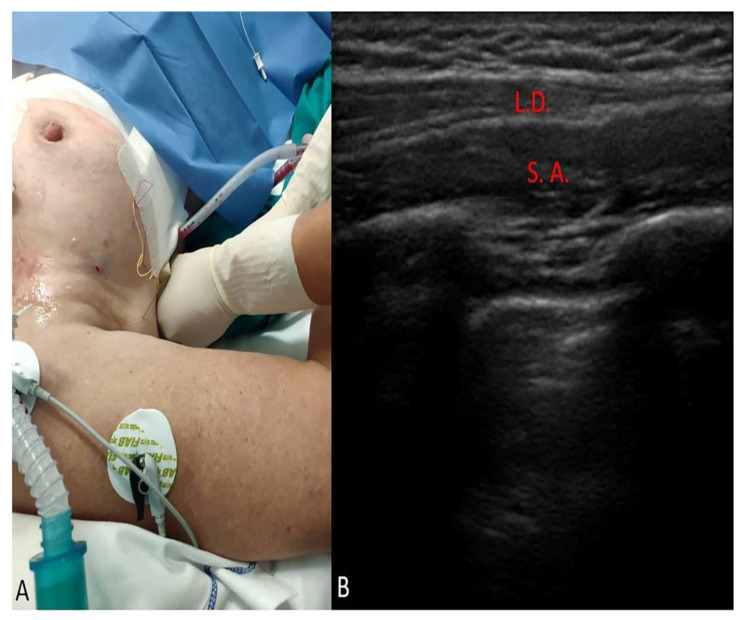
SAP block: (A), intraoperative positioning for the realization of the block. (B) ultrasound imaging scan of the inter-fascial plane deep to the anterior serratus muscle at 5th rib level in the median axillary line; L.D.: latissimus dorsi muscle; S.A.: anterior serratus muscle.
